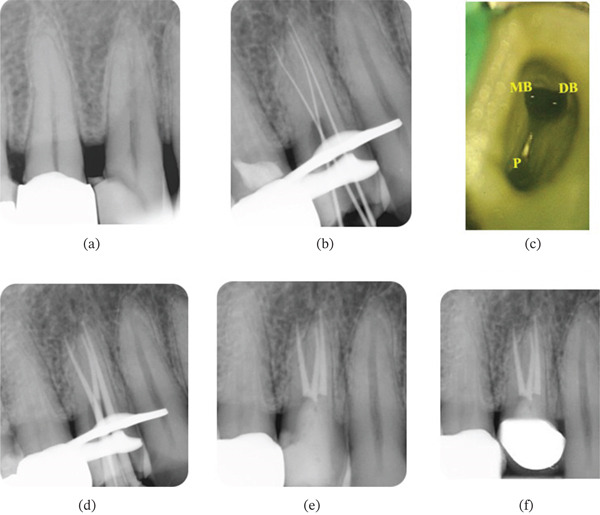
(a) Preoperative radiograph of 14; (b) working length radiograph; MB = 19 mm, DB = 18.5 mm, and P = 20.5 mm; (c) access cavity with a T‐shaped configuration showing three orifices under magnification; (d) master cone radiograph; (e) post obturation and core build up radiograph; and (f) follow‐up radiograph at the end of 1 year with PFM full coverage crown.
